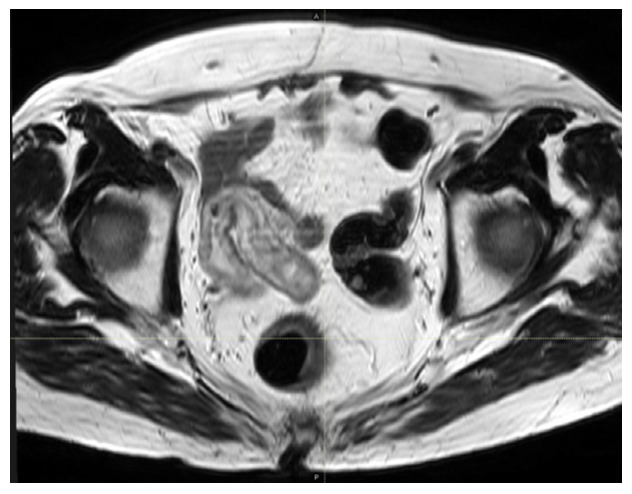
Pelvic MRI scan performed 6 weeks after the interruption of the radiochemotherapy. Compatibly with the different exam, a moderate thickening of the ileal walls can still be noticed.
